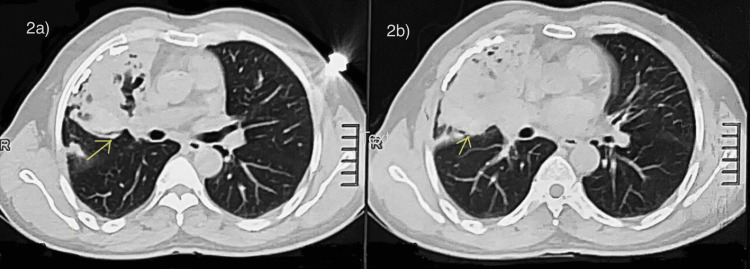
(a, b) CT of the chest showing minimal pneumonitis with fibrotic bronchiectatic strands on the lateral segment of the right middle lobe.
